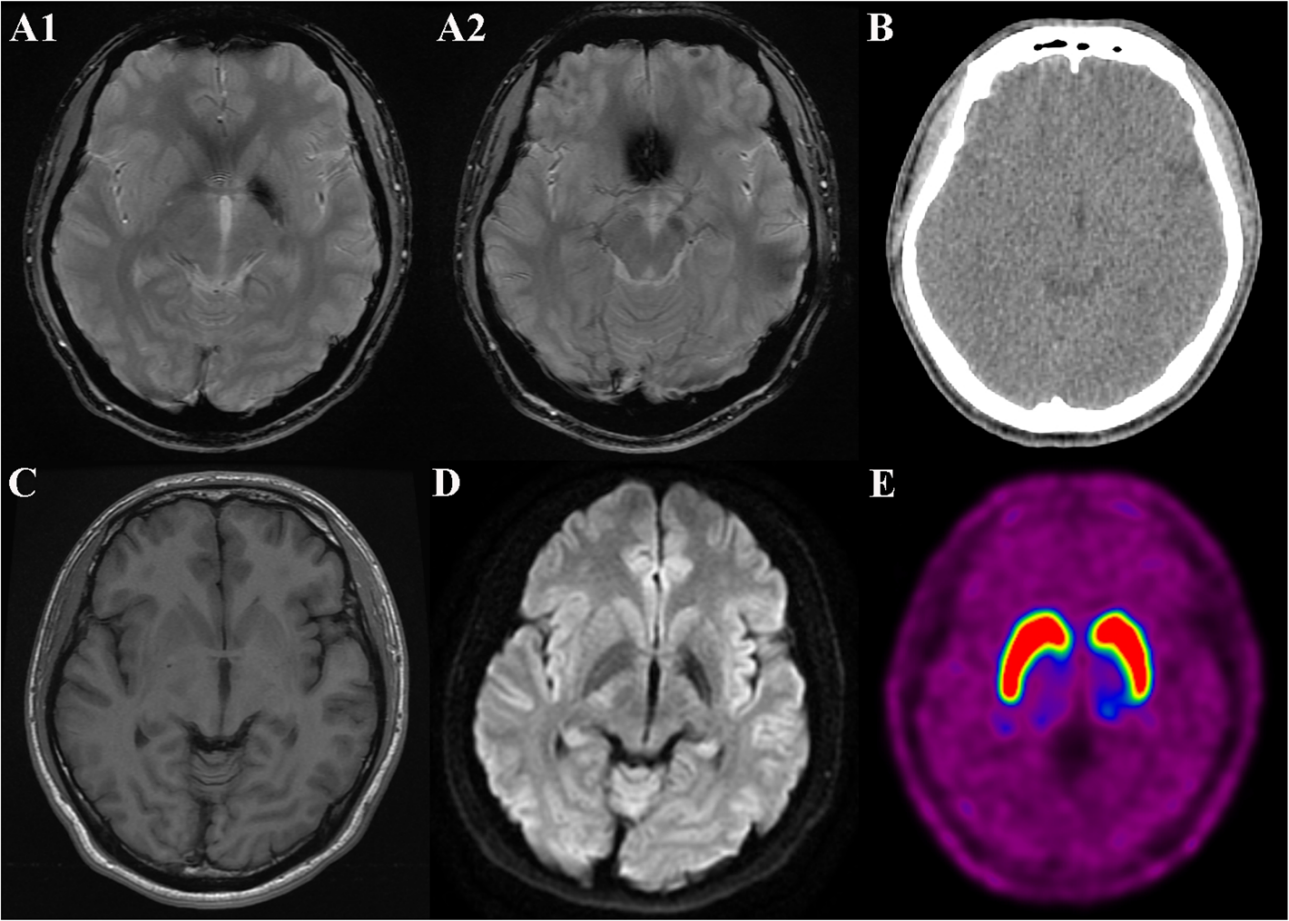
Neuroimaging of the patient. T2*-weighted gradient-echo sequences reveal the presence of hypointensities within the left globus pallidus (a1) and left substantia nigra (a2). The left globus pallidus shows no signal change on computed tomography scan (b) or T1-weighted image (c), while a low signal abnormality is seen on diffusion-weighted imaging (d). The [^18^F] N-(3-fluoropropyl)-2β-carbon ethoxy-3β-(4-iodophenyl) nortropane positron emission tomography scan shows no pathological striatal alterations (e)
